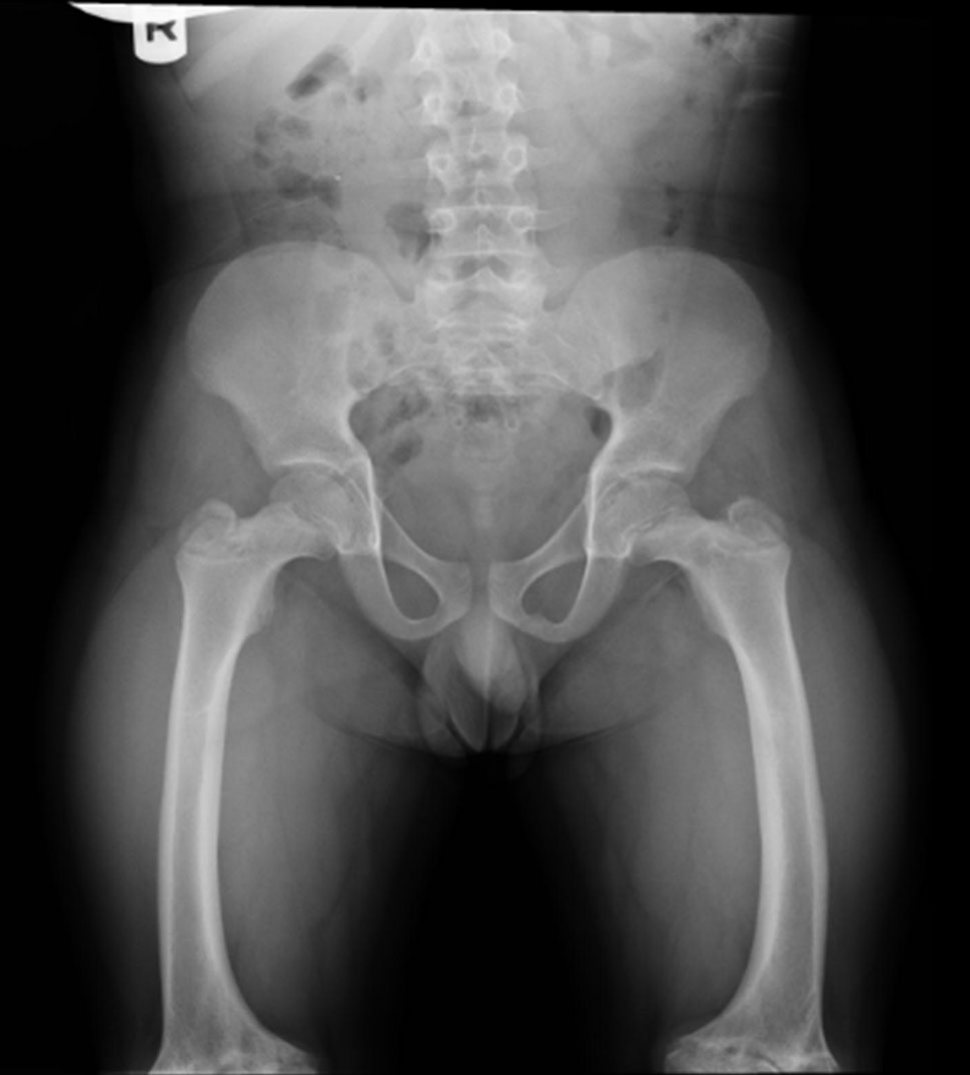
Anteroposterior radiograph demonstrating coxa vara and femoral bowing
